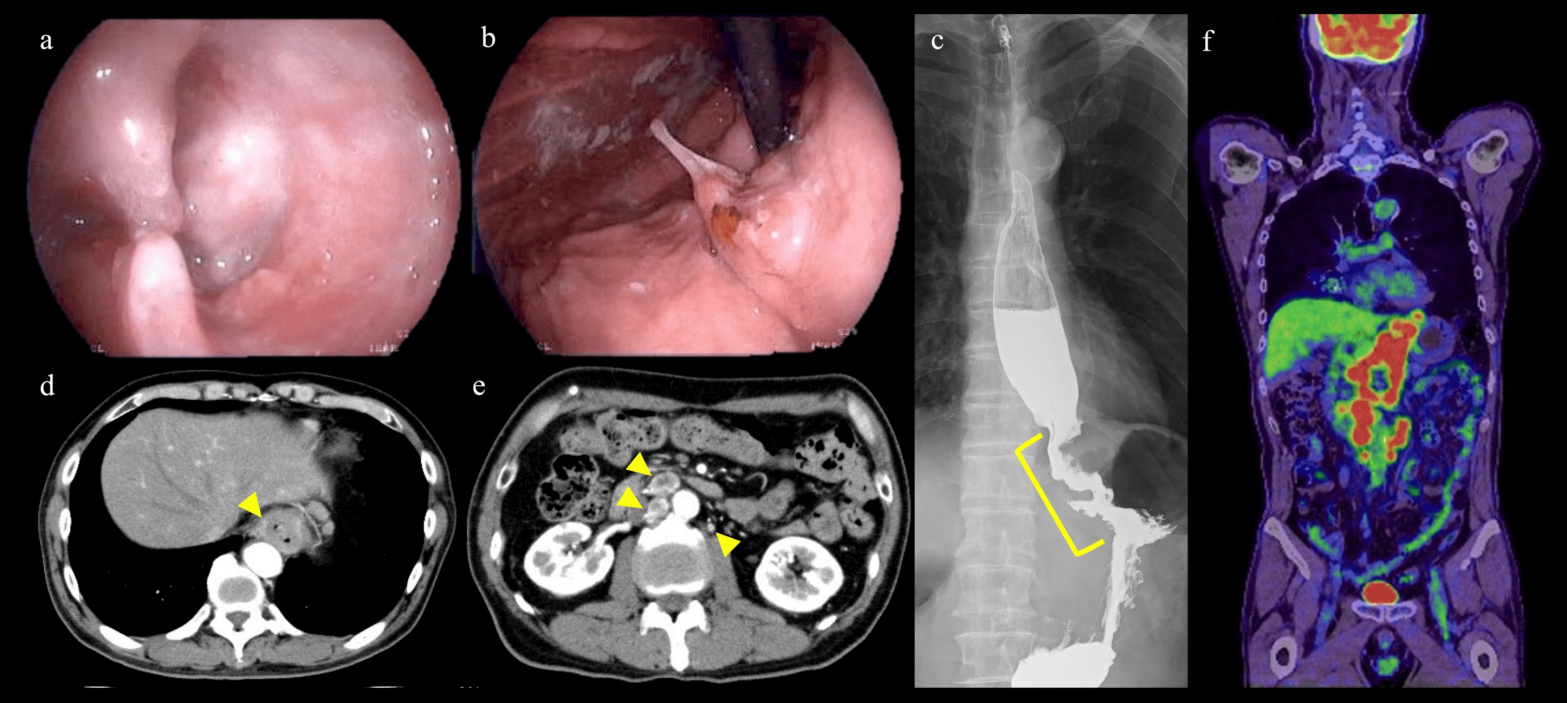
Preoperative images. (a, b) Upper gastrointestinal endoscopy showing an ulcerative and infiltrative advanced esophageal tumor with circumferential stenosis extending from the abdominal esophagus to the cardia. (c) Upper gastrointestinal tract radiography showing an irregular mucosa with stenosis of 5 cm in length from the abdominal esophagus to the cardia. (d, e) CT showing esophageal wall thickening extending from the abdominal esophagus to the hilum, along with enlarged lymph nodes in the lower para-esophagus, cardiac region, lesser curvature of the stomach, and para-aortic region (yellow arrowhead). (f) PET-CT showing abnormal fluorodeoxyglucose (FDG) accumulation in the primary lesion, and lymph nodes in the lower para-esophagus, cardiac region, lesser curvature of the stomach, and para-aortic region. No obvious distant organ metastasis was detected.
